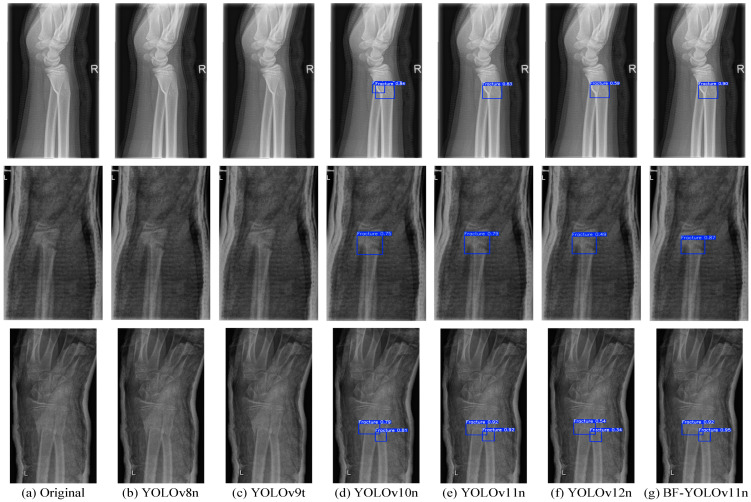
Comparative Effectiveness of Mainstream X-ray Fracture Detection Models. (a) Untreated original image; (b) Image detection using YOLOv8n; (c) Image detection using YOLOv9t; (d) Image detection using YOLOv10n; (e) Image detection using YOLOv11n; (f) Image detection using YOLOv12n; (g) Image detection using BF-YOLOv11n.
